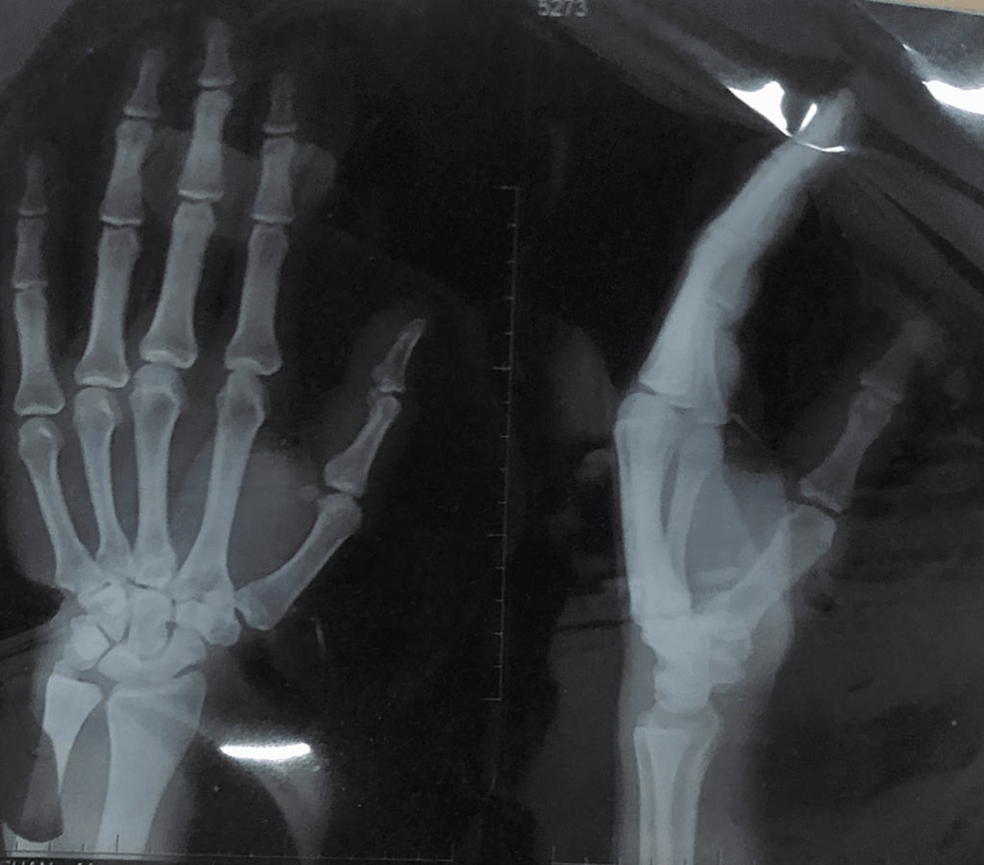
X-ray hand anteroposterior and lateral views showing no bony involvement with mild soft tissue swelling of the left thumb
